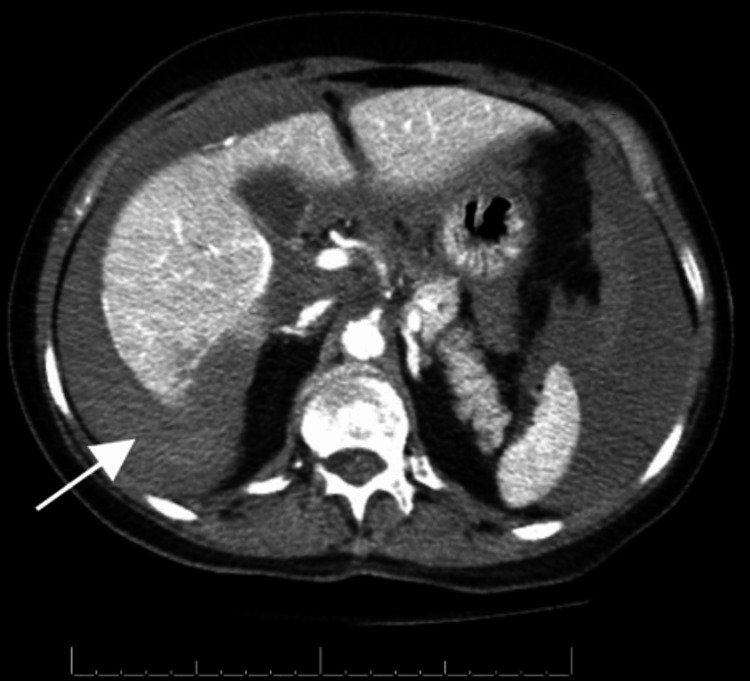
Representative axial portal venous phase CT image on admission showing hemoperitoneum (white arrow). CT: computed tomography.
